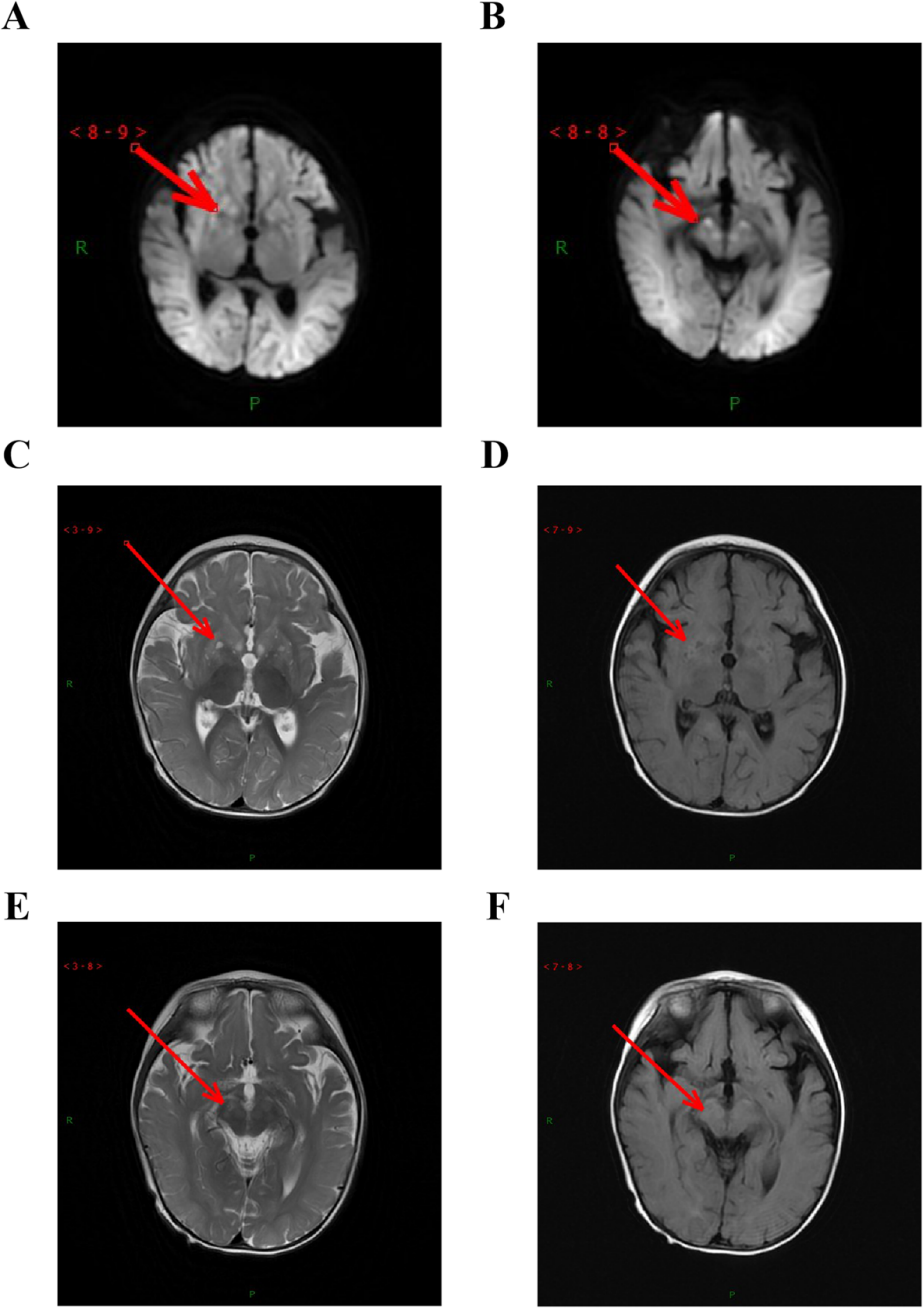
The MRI assay of the patient. (a-b) DWI image; (c-d) T2 image;(e-f)T2 FLAIR image
